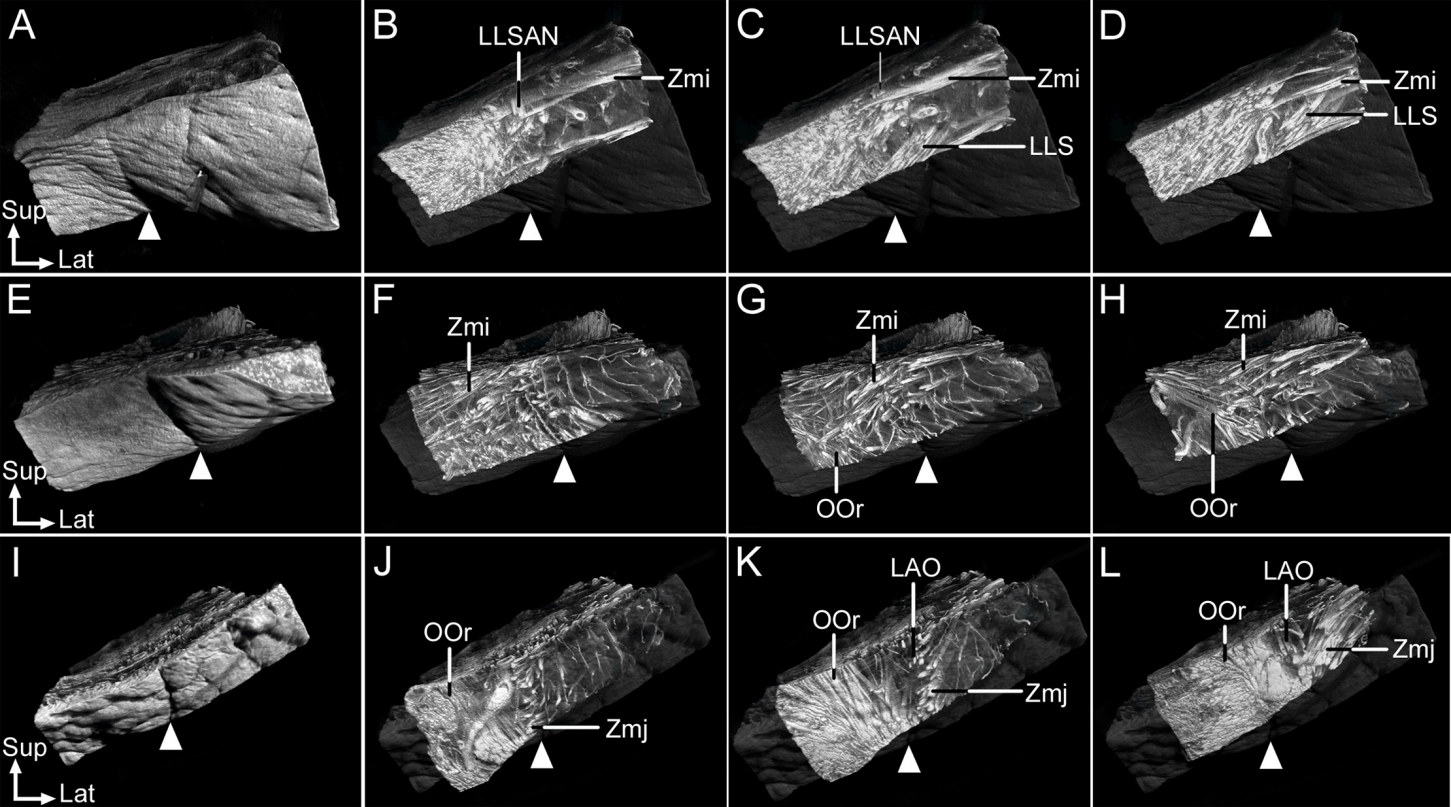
Heights and spatial relationships of the facial muscles acting on the NLF from the skin to the deeper area as revealed by microcomputed tomography. (A–D) The NLF in its medial half (arrowhead) (A). (B) Beneath the skin of the NLF, LLSAN and Zmi fibers inserted into the dermis of the NLF and adjacent to the NLF. (C) At the deeper site of the NLF, the LLSAN, LLS, and Zmi fibers interdigitated together in different directions. (D) At the still-deeper site, more LLS fibers were found with the Zmi fibers. (E–H) The NLF in its middle third (arrowhead) (E). (F) Beneath the skin of the NLF, the Zmi fibers were found before their insertions into the dermis of the NLF and adjacent to the NLF. (G) At the deeper site, more Zmi fibers and some OOr fibers were found. (H) At the still-deeper site, the Zmi fibers intermingled with the lateral margin of the OOr. (I–L) The NLF in its lateral third (arrowhead) (I). (J) Beneath the skin of the NLF, the lateral margin of the OOr and some Zmj fibers were found. (K) At the deeper site, more Zmj and OOr fibers blended with the levator anguli oris muscle. (L) At the still-deeper site, more OOr and Zmj fibers were found with the LAO. At all levels of the NLF, the location of the NLF (arrowheads) corresponded with the lateral margin of the OOr where the LLSAN, LLS, Zmi, and Zmj intermingled and blended with that margin. The skin was made transparent to reveal the deeper areas so that the location of the NLF and the facial muscles beneath it could be compared. Serial images of the facial muscles beneath the NLF are presented in an online video. Lat, lateral; LAO, levator anguli oris muscle; Sup, superior.
